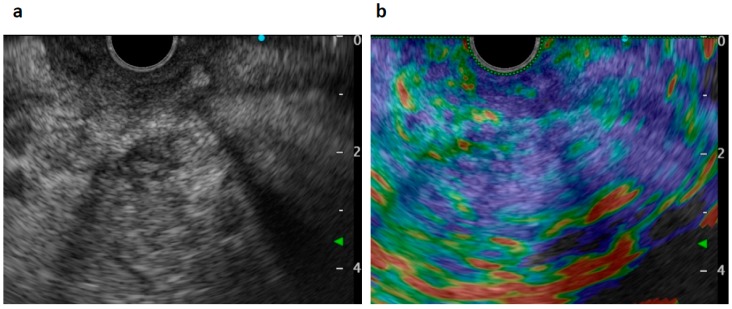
Endoscopic ultrasonography (EUS) views. A heterogeneous low-echoic mass was seen by scanning from the duodenal bulbs (a). Elastography showed a heterogeneously hard mass lesion at the pancreas head (b).
